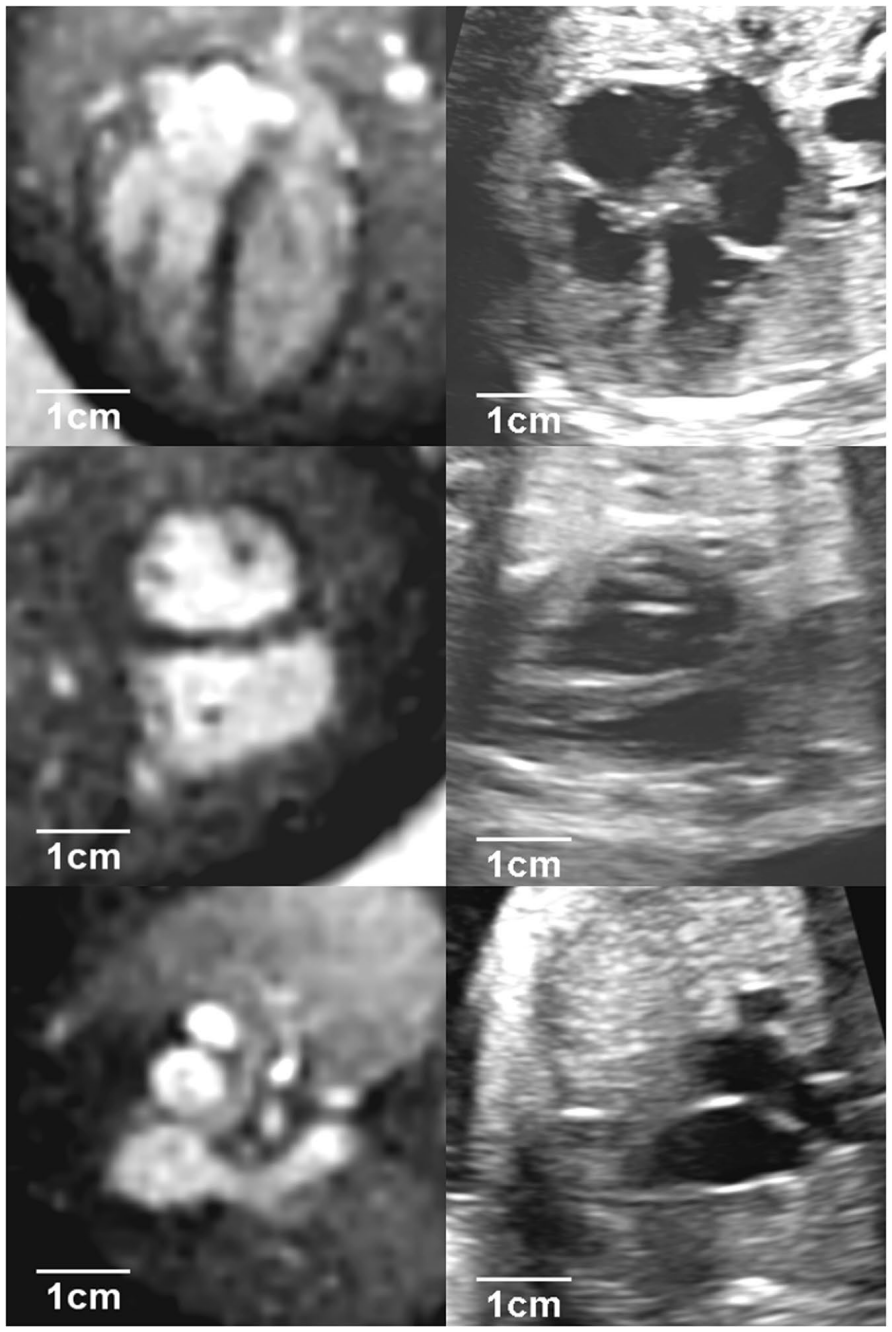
Comparison of MR (left) and echocardiographic (right) images. Top row: 4-chamber view; middle row: short-axis view; bottom row: 3-vessel view.
